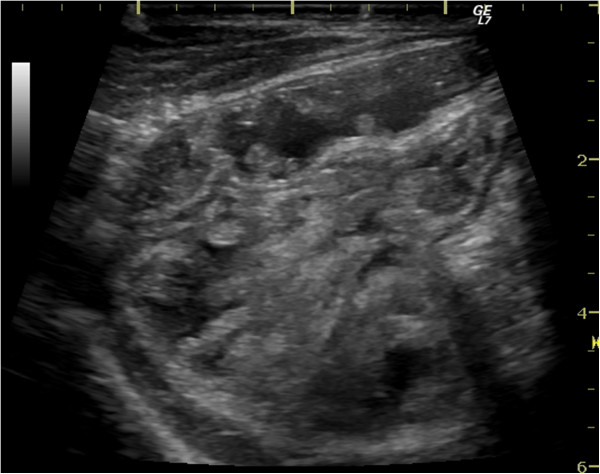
Abdominal ultrasound – intestinal loops with enlarged, hyperechoic mesentery.
